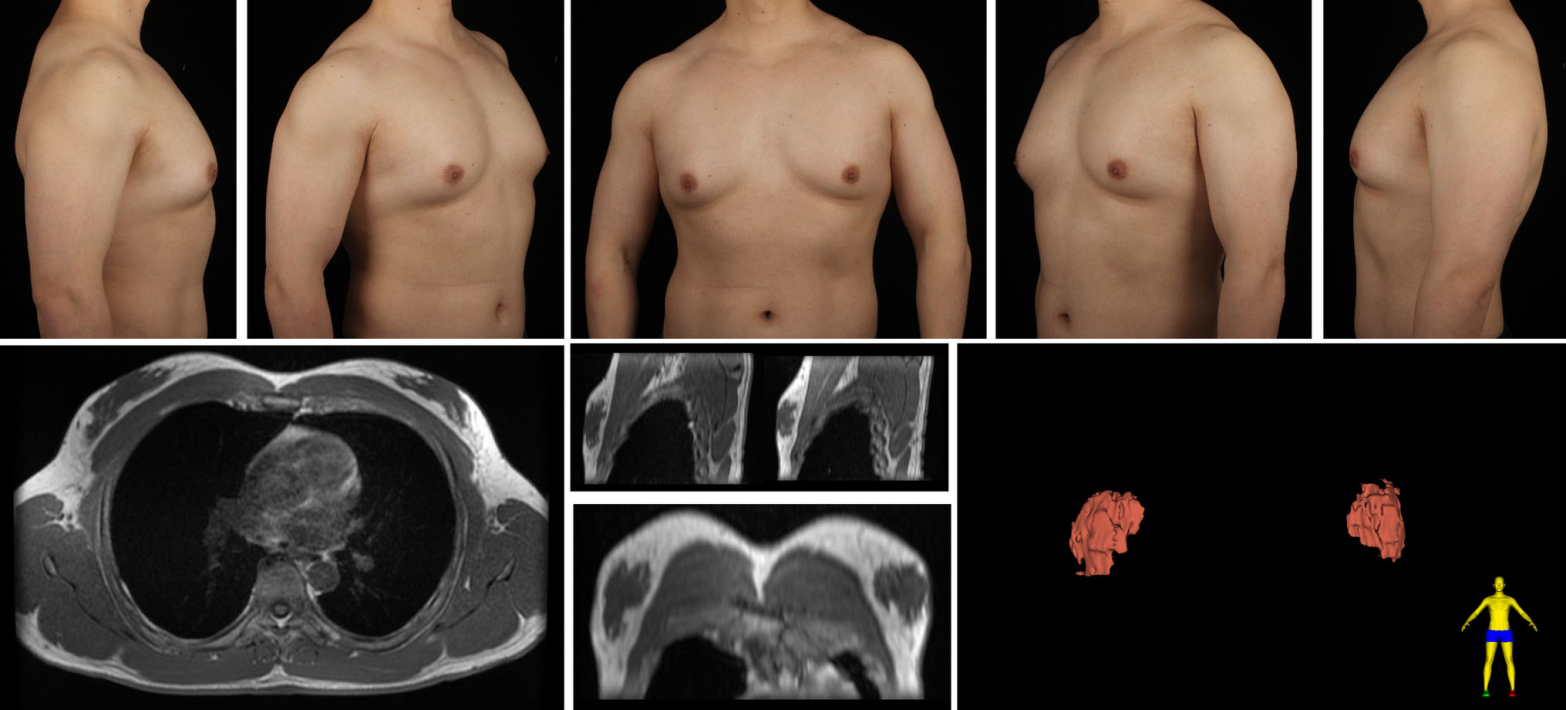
Type 2C gynecomastia. Preoperative chest appearance (upper) and breast MR images (lower) of a 42-year-old man with type 2C gynecomastia
